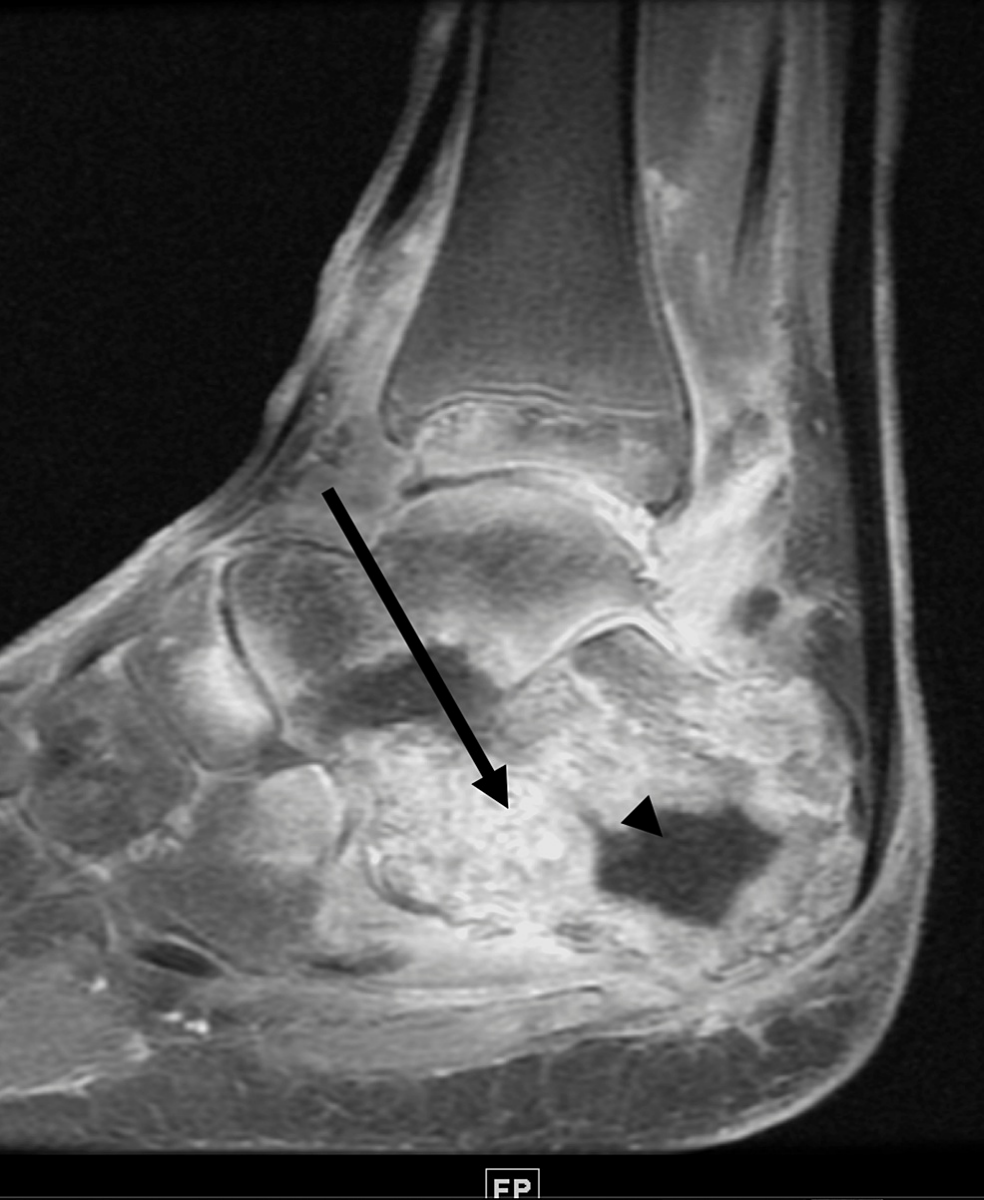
Contrast-enhanced MRI of left ankle joint in Case 1 demonstrated intense calcaneal bone marrow edema and enhancement on post-contrast administration (long arrow) and a focal area of non-enhancement suggestive of necrotic bone marrow (arrow head).
